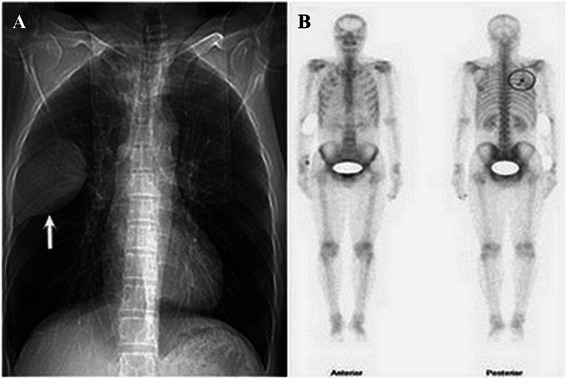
Preoperative chest roentgenogram and bone scanning examinations. Chest roentgenogram showing a solitary expanding lesion in the right sixth rib (*arrow*, a). Abnormally increased uptake of radioisotope in the right sixth rib on a bone scan (b)
